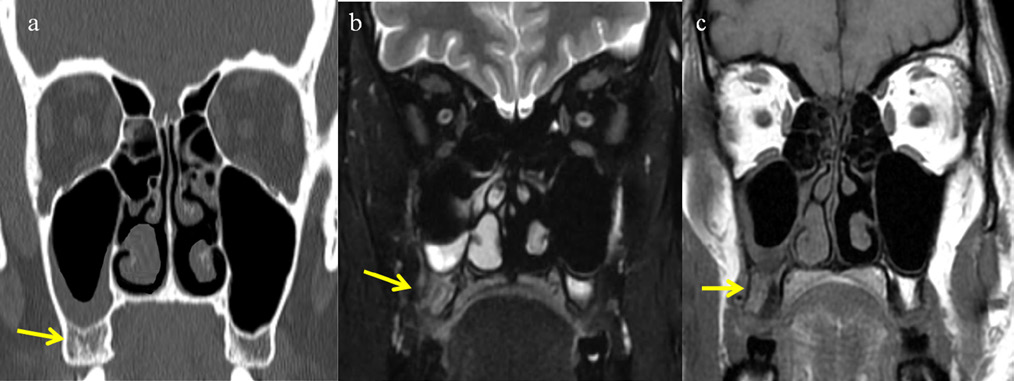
Coronal CT and MRI images of early mucormycosis showing bone marrow infiltration. (a) Coronal CT (Bone window) showing mucosal thickening in right maxillary sinus. Bone involvement is not clearly appreciated. Coronal T2FS(b) and coronal T1W (c) images showing abnormal hyperintensity (arrow) in right alveolar process of maxilla (b) and replacement of normal fatty marrow (arrow) compared to the normal opposite side (c).
